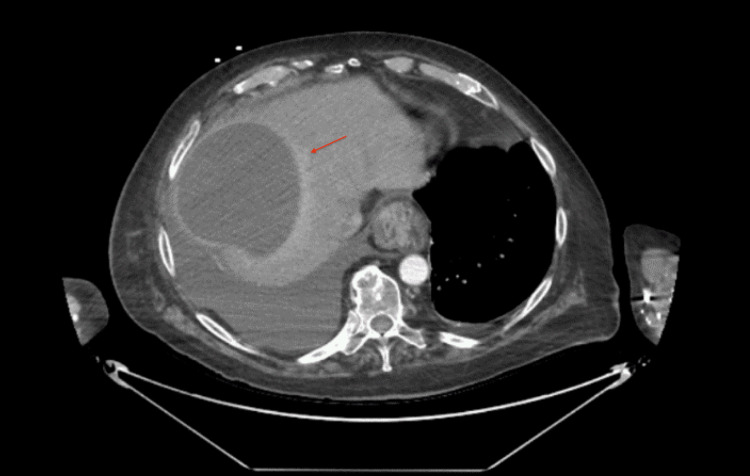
Triple-phase liver CT with hypodense right hepatic lobe lesion with ring enhancement on the late arterial phase.
